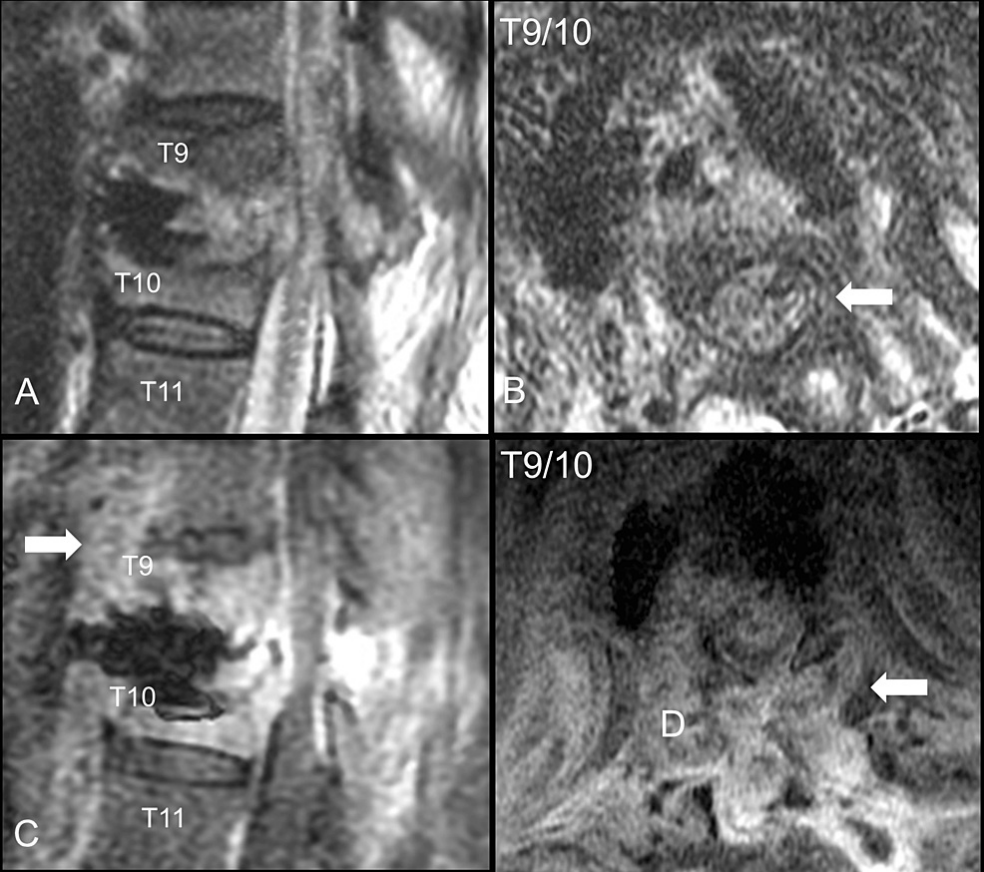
Post-laminectomy magnetic resonance image of the thoracic spine. (A) T2 without contrast, sagittal view. Abnormal soft tissue around the epidural region with spinal stenosis. (B) T2 without contrast, axial view. Dura was not compressed (arrow). (C) T1 with contrast, sagittal view. Paraspinal-enhanced soft tissue was identified (arrow). (D) T1 with contrast, axial view. Paraspinal-enhanced soft tissue was identified (arrow).
